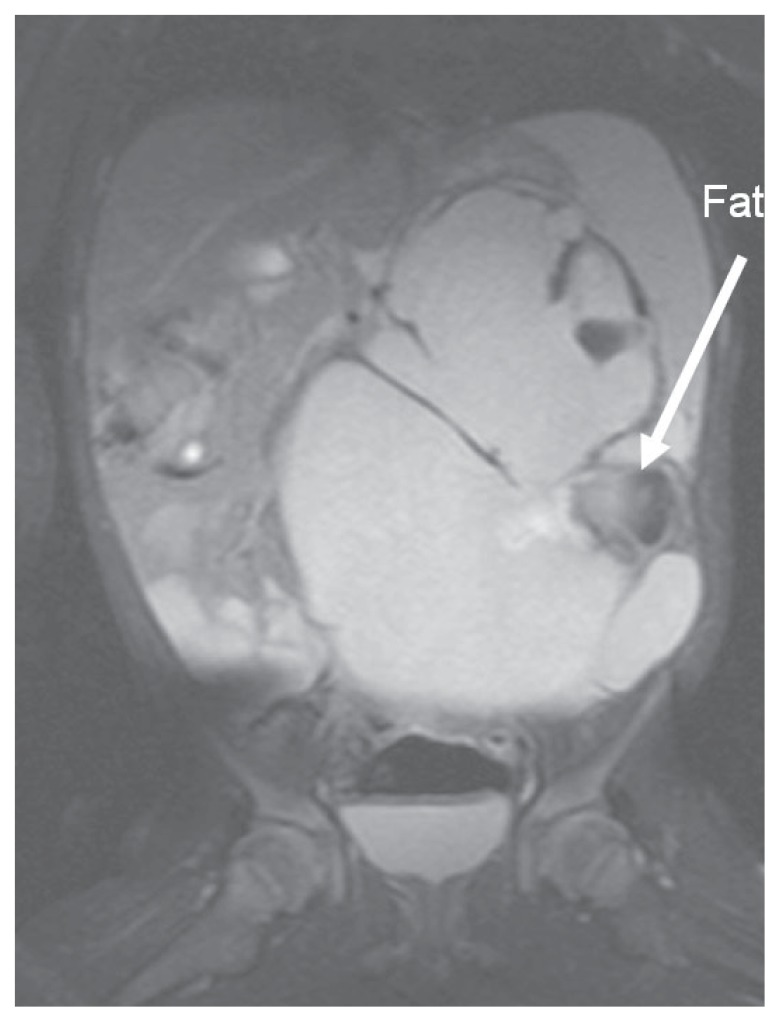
Zystic-solid teratoma in the left abdomen (case 2; MRI, T1, coronal).
